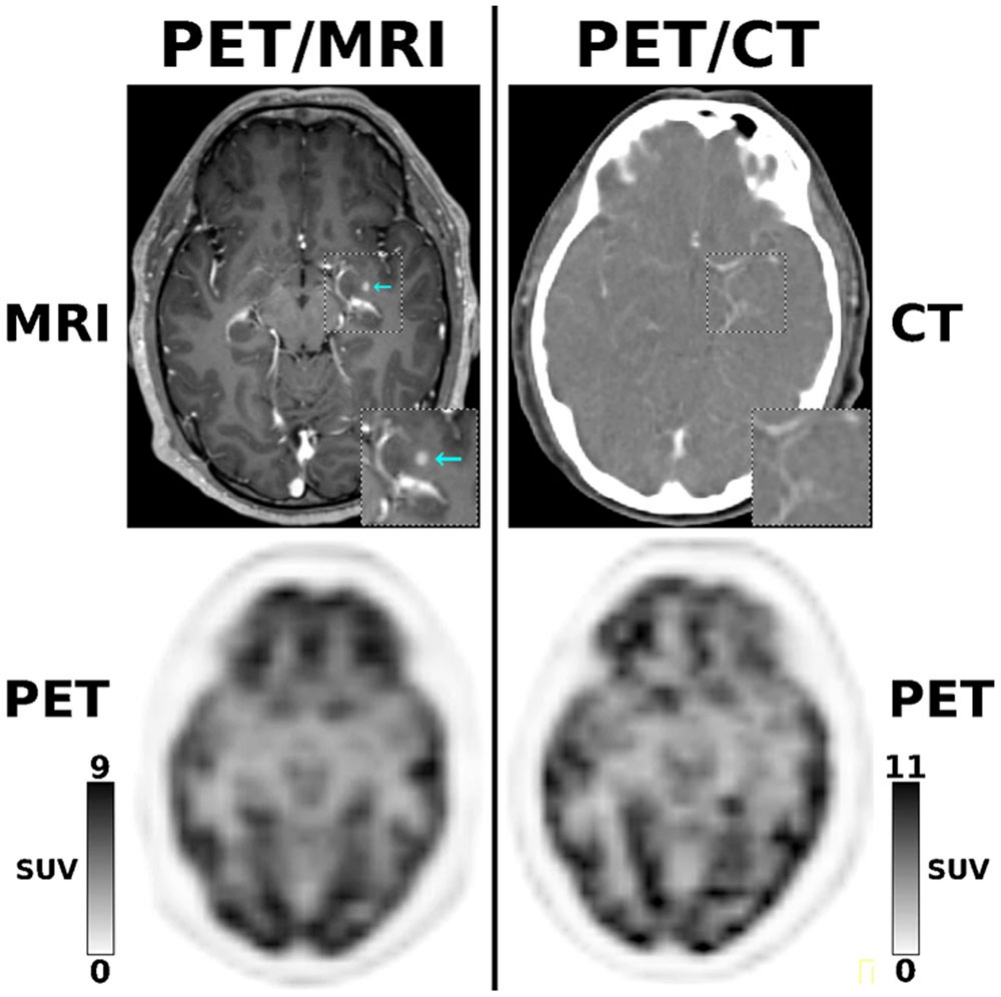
Imaging of a 61‐year‐old non‐small cell lung cancer (NSCLC) stage IV patient for staging before nivolumab. [18F]FDG‐PET is negative on both PET/MRI and PET/CT, but contrast‐enhanced MRI in PET/MRI identifies a small left hippocampal metastasis (cyan arrow), missed by contrast‐enhanced CT in PET/CT, warranting RT.^65^ Abbreviations: CT, computed tomography; MRI, magnetic resonance imaging; PET, positron emission tomography. [Color figure can be viewed at wileyonlinelibrary.com]
